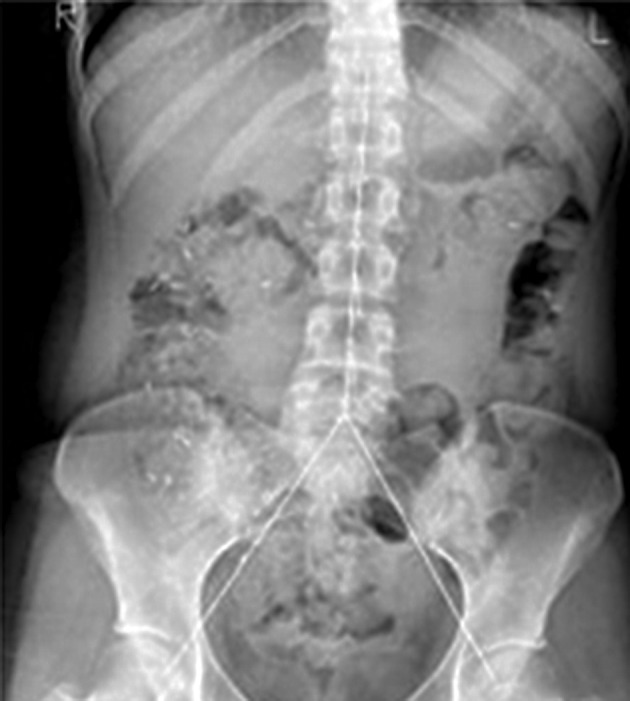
The plain Abdominal x-ray is Divided into 3 Segments and Radiopaque Markers are Counted in Each Segment
